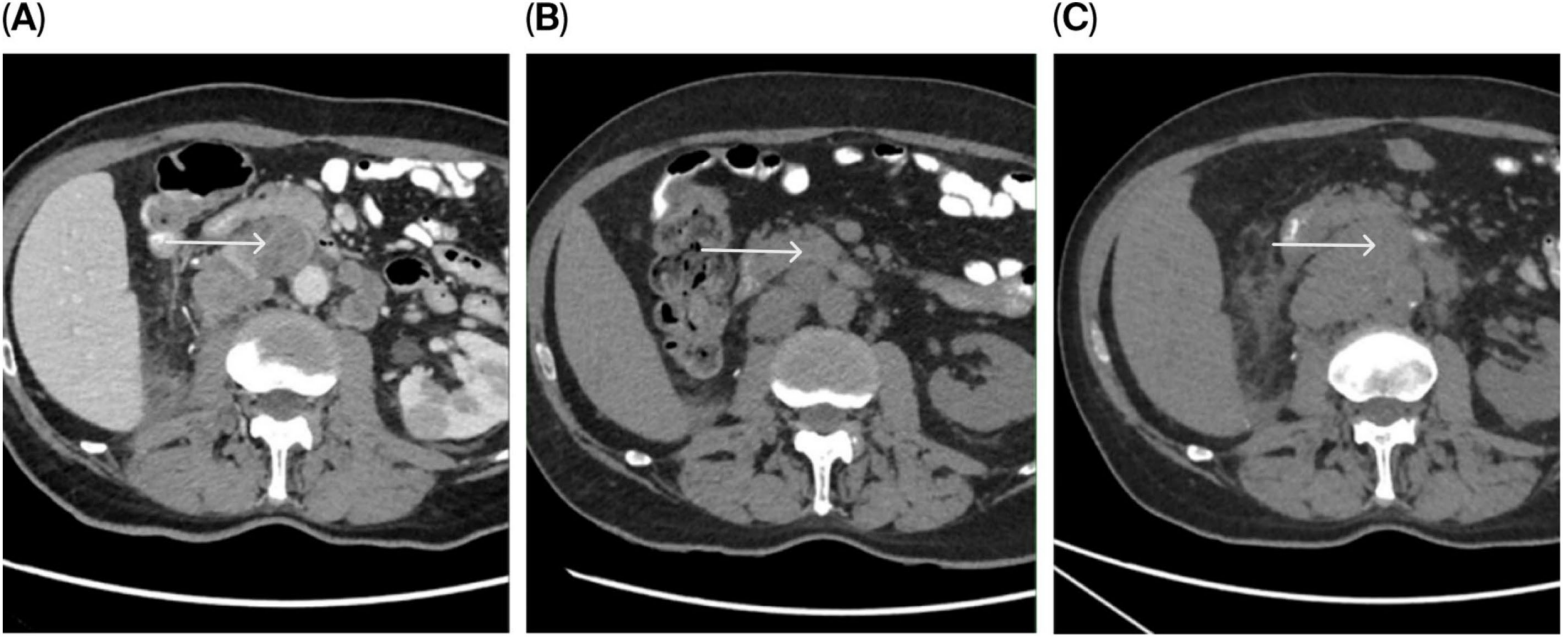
Immune response assessment by iRECIST on CT. (A) Baseline imaging was performed on 13 January 2025. (B) Follow-up was on 15 April 2025, showing a decrease in target retroperitoneal lesions consistent with iPR (the white arrow). (C) On 15 July 2025, an increase in tumor burden meeting RECIST 1.1 progression criteria was observed; according to iRECIST, this was categorized as *iUPD*.
